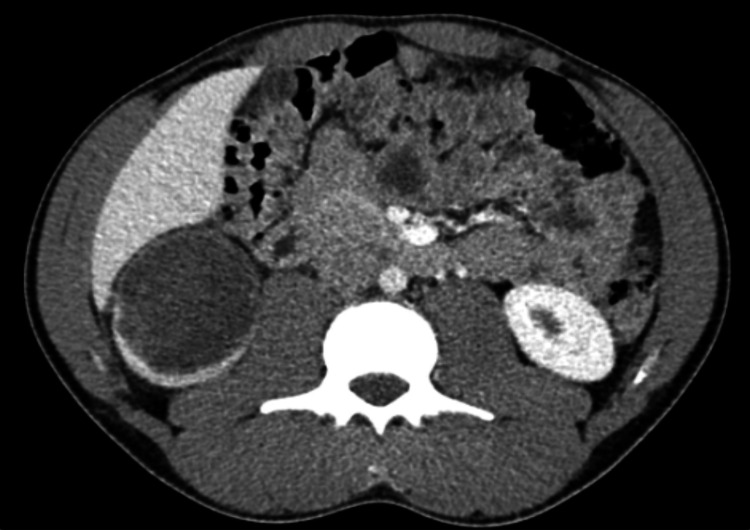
Abdomino-pelvic CT scan Cystic right lower renal cortical mass with right hypoenhancing right retroperitoneal lymph node suspicious for malignancy. Increased skeletal muscle surface area was identified in retrospect: the psoas muscles and erector spinae muscles are hypertrophic.
